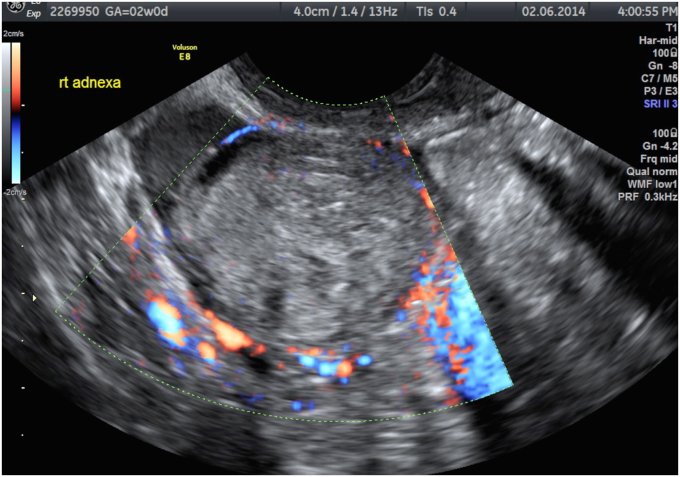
Ultrasound using color Doppler indicates a right adnexal mass with a “ring of fire” vascularity. Reprinted from content created by Alexandra Stanislavsky from Radiopaedia.org, rID:47667, under CC BT–NC–SA 3.0 license (https://creativecommons.org/licenses/by-nc-sa/3.0).
